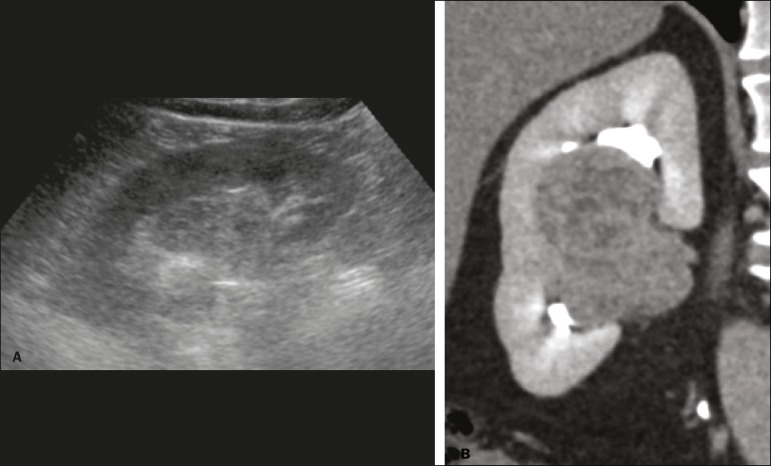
Renal masses missed on initial ultrasound and detected on subsequent
